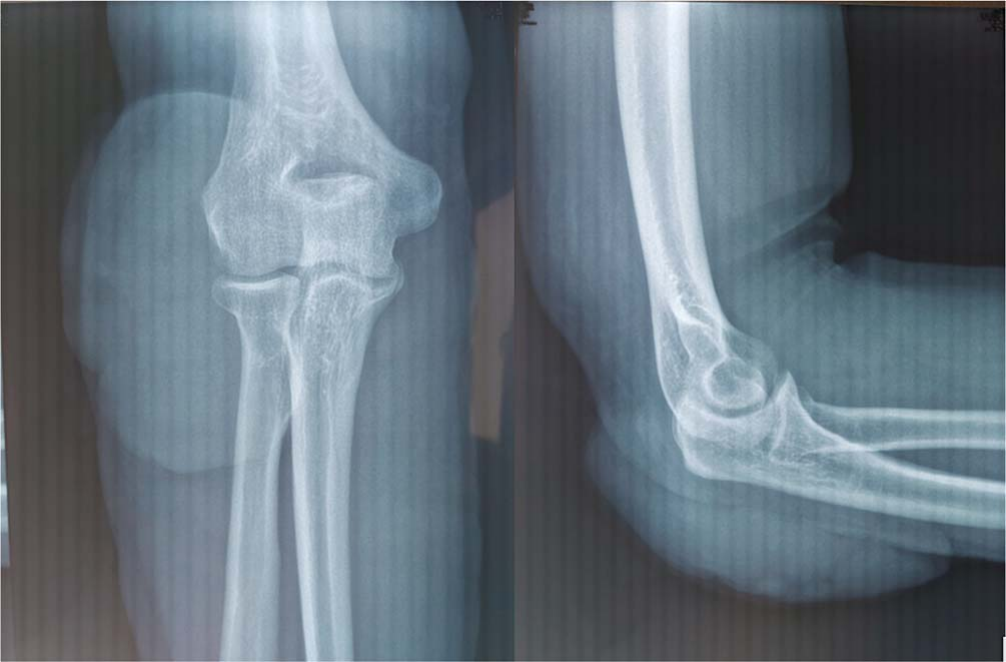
Front and side X-rays of the elbow.
